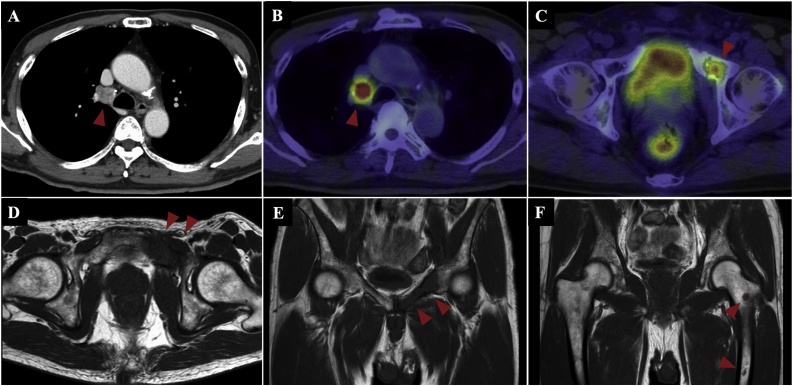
Imaging of primary and metastatic lesions. Arrowheads indicate the tumor. (A) Contrast-enhanced computed tomography reveals mediastinal lymphadenopathy. (B) This lymph node demonstrates high fluorodeoxyglucose uptake on positron emission tomography. (C) A high level of fluorodeoxyglucose accumulation is observed in the pubic bone. (D) T1-weighted magnetic resonance imaging shows multiple tumors of the pubic bone and femur, (E) coronal section, and (F) sagittal section.
